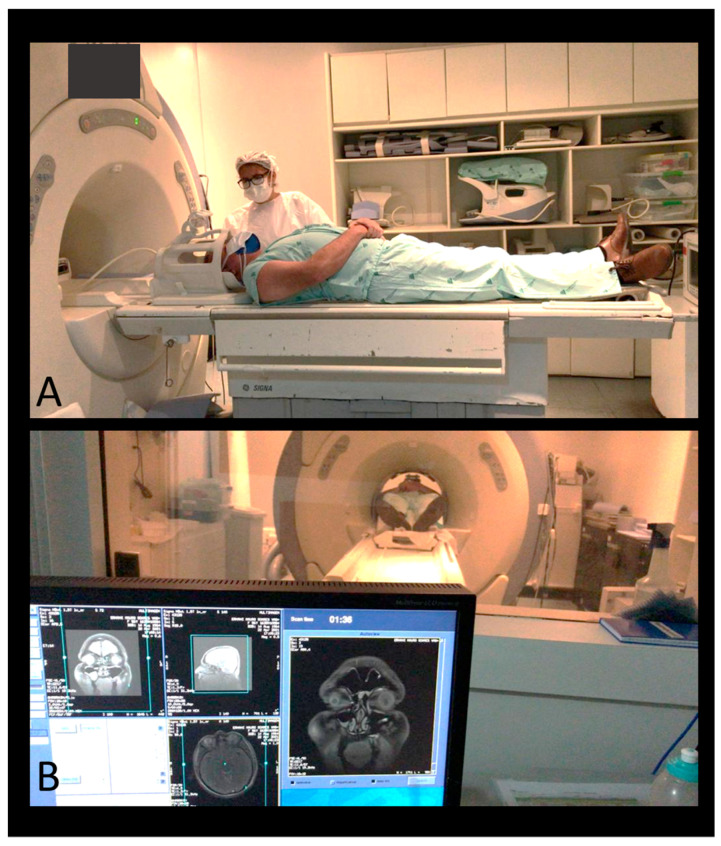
Moments during the performance of MRI on the brain of a patient with COVID-19 (A) Patient being positioned in the MRI machine with the brain coil having been placed. (B) Photograph of the MRI Control Room during the examination and the acquisition of the brain magnetic resonance images of a patient with COVID-19.
